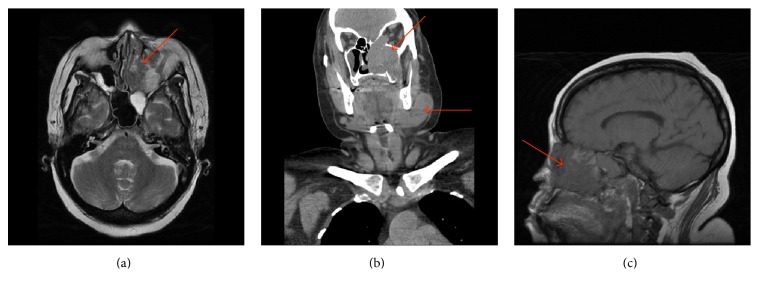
((a) (axial), (b) (coronal), and (c) (sagittal)) CT scan of the neck showing extensive lymphadenopathy, left greater than right, and extensive soft tissue involving the left nasal cavity and multiple paranasal sinuses wit invasion into the left orbit and left infratemporal fossa.
